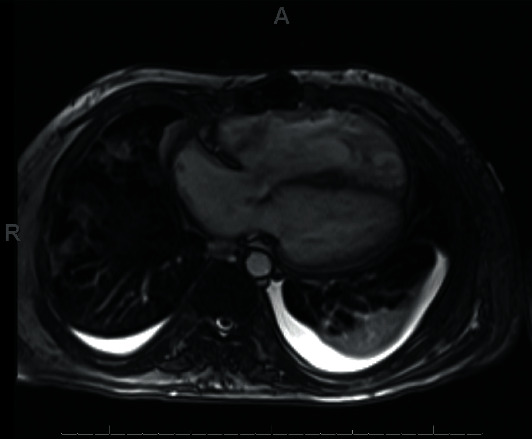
Cardiac magnetic resonance imaging demonstrating marked right ventricular enlargement.
